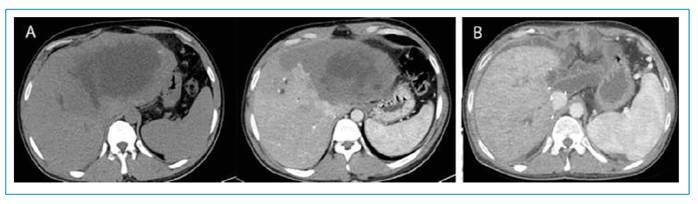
Axial non-contrast and contrast-enhanced computed tomography (CT) images (A) show a hypodense mass lesion in the left lobe of the liver before transplantation. Axial contrast-enhanced CT imaging after liver transplantation (B).
